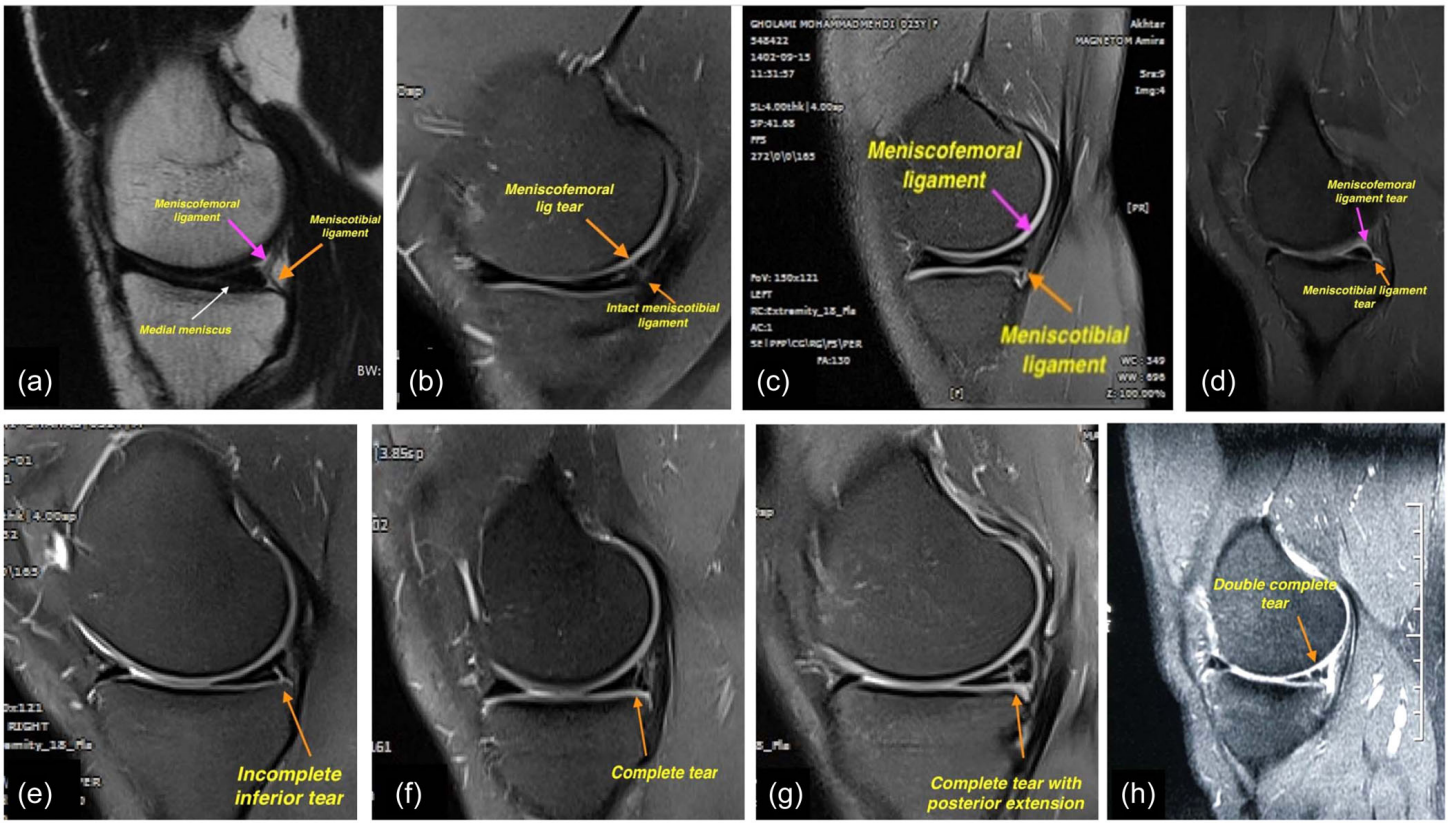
Magnetic resonance imaging (MRI) images of medial meniscal ramp lesions (RLs). (a) Normal meniscus, (b) meniscofemoral tear, (c) meniscotibial from meniscus, (d) both meniscofemoral and meniscotibial from meniscus, (e) incomplete inferior, (f) complete single, (g) complete single with posterior extension, (h) double complete.
